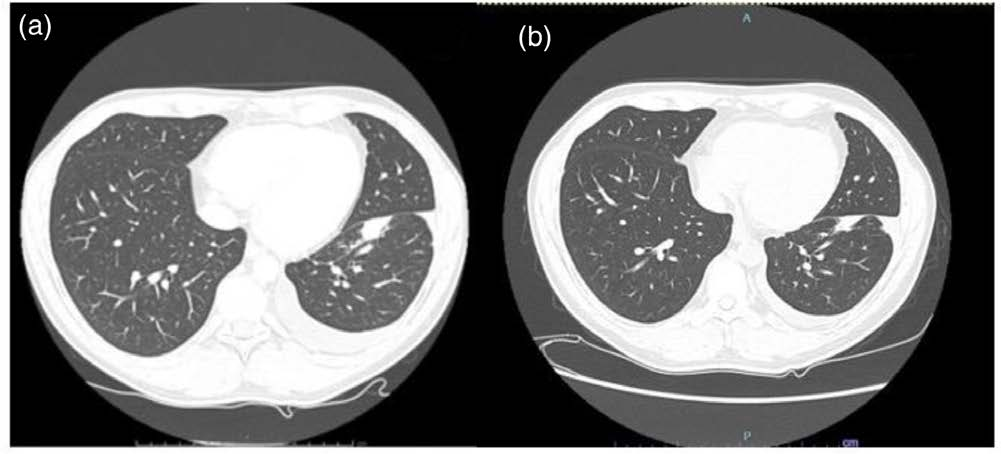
Computed tomography (CT) scans of primary nodule at the beginning of treatment with lorlatinib (a—March 2021) and after 36 months (b—January 2024) showing stable lung disease.
